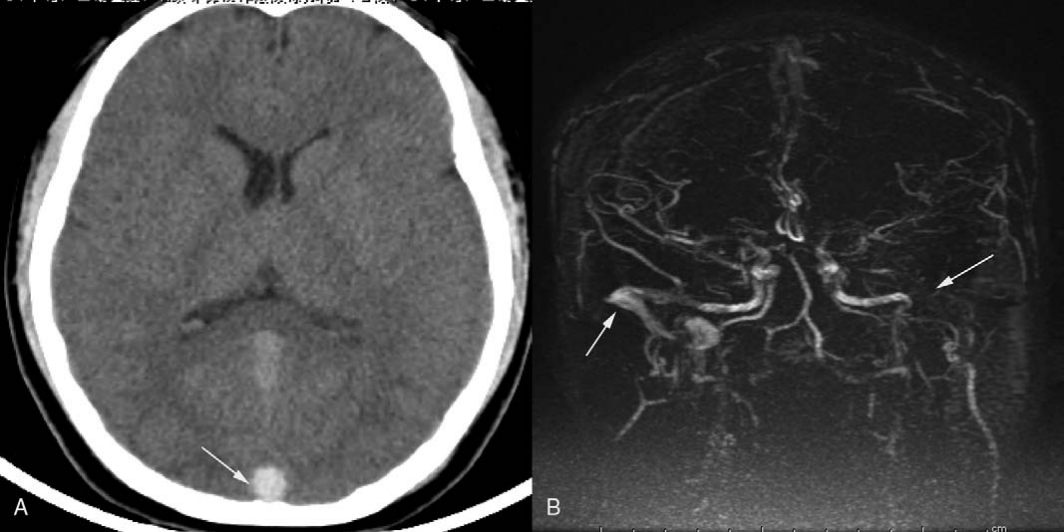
A thrombus sign in the SSS (white arrow) observed on CT; MRV showing a normal flow signal in the right transverse sinus and sigmoid sinus, but non-visualization of the left transverse and sigmoid sinus (white arrows). CT = computerized tomography, MRV = magnetic resonance venography, SSS = superior sagittal sinus.
